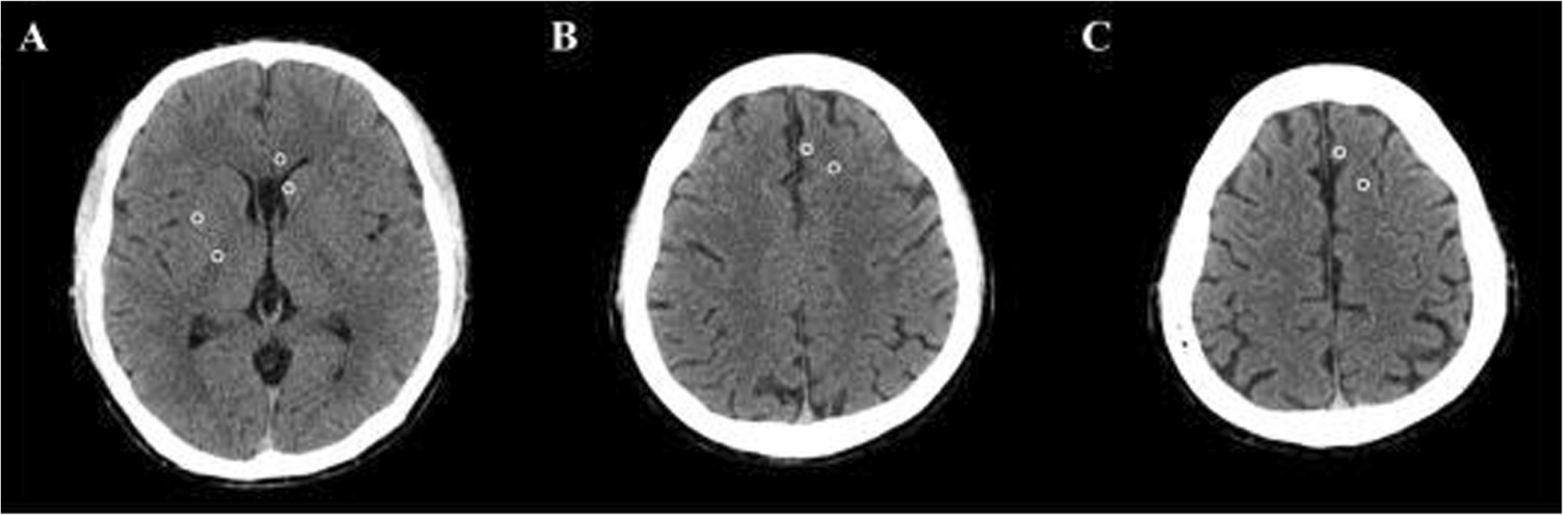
Brain computed tomography images showing measurements in Hounsfield units at the basal ganglia level (left), the centrum semiovale level (middle), and the high convexity level (right). The circular regions of interest (10 mm2) are positioned in the caudate nucleus, putamen, posterior limb of the internal capsule, and genu of the corpus callosum at the level of the basal ganglia and are positioned in the medial cortex and medial white matter at the centrum semiovale and high convexity levels.
